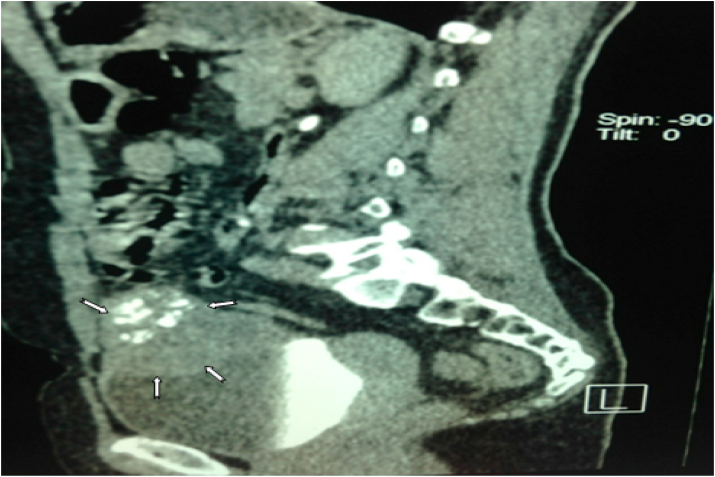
Abdomino-pelvic CT revealed a bladder tumor process budding at the expense of the left anterolateral wall with exophytic development and site of calcifications.
